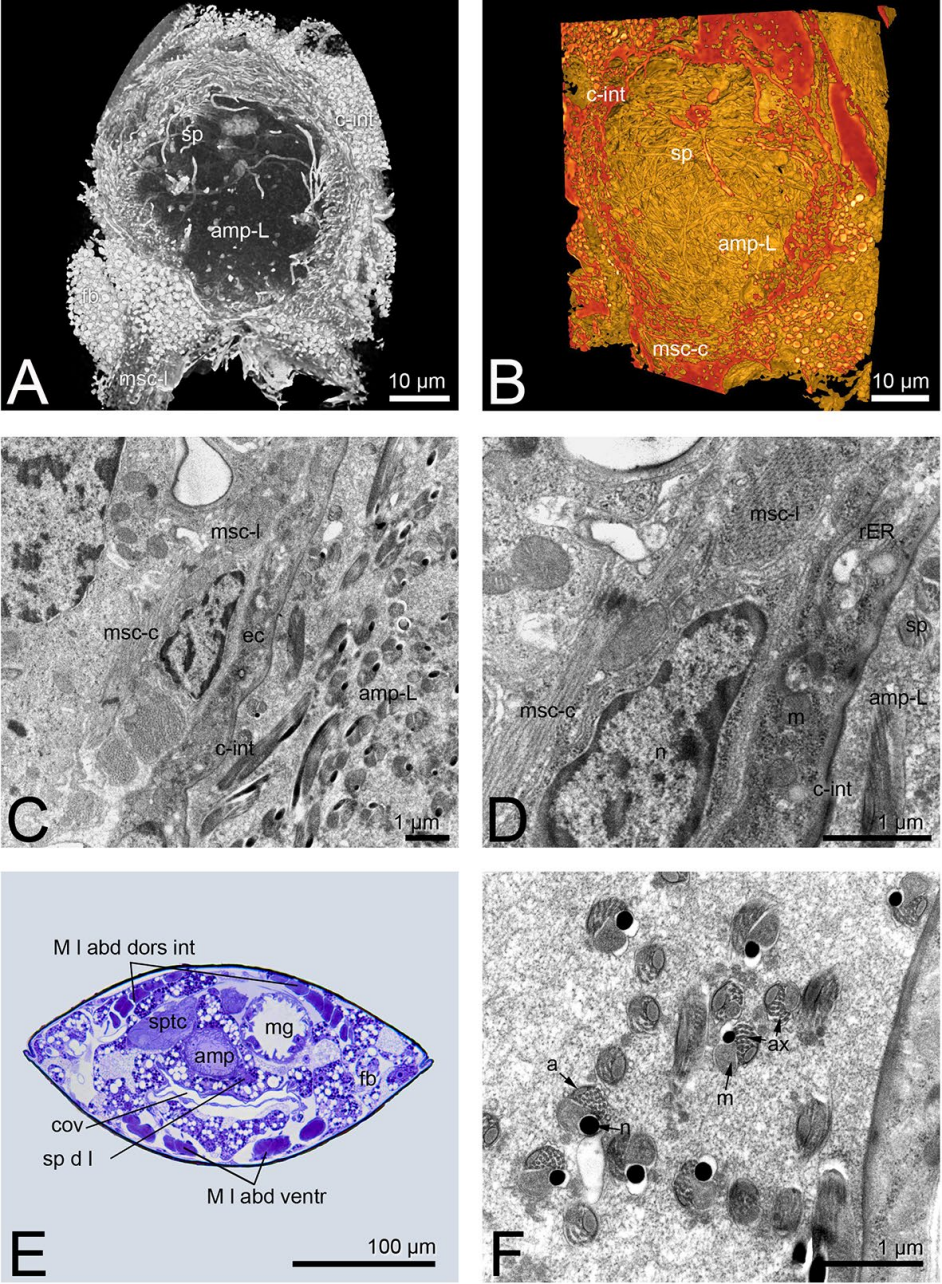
Suocerathrips linguis*, (A) ampoule, nano-CT frontal section; (B) ampoule filled with sperm, nano-CT oblique view; (C) border area of the ampoule with the lumen and the three-layered epithelial structure, (D) epithelial layer consisting of epithelial cells, muscle cells and a connection layer to the fat body; (E) location relationship of ampoule and spermatheca within the body cavity, transverse section; (F): close up of spermatozoa ultrastructure within the ampoule.
